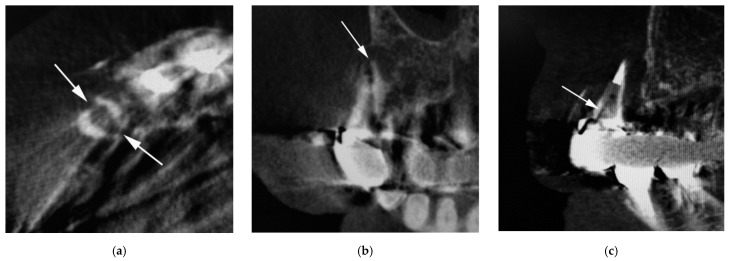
Cone-beam computed tomography (CBCT) image of vertical root fractures in endodontically treated teeth (bucco-lingual fracture). (a) Axial image; (b) cross-sectional multiplanar reformation (MPR) image; and (c) parasagittal MPR image. Arrow indicates part of root fracture.
